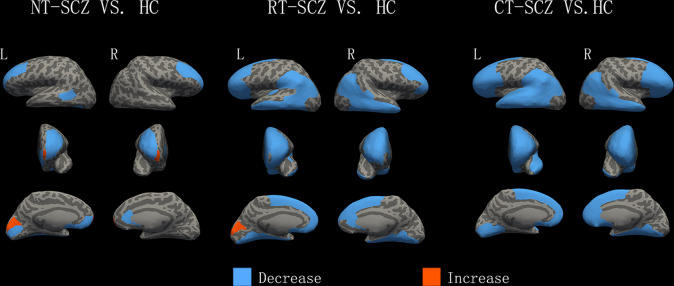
Differences in GM between patients with chronic schizophrenia and healthy controls. Greater cortical volume, surface area, or thickness in patients than in comparison subjects are indicated by red/warm color, and lower volume, surface area, or thickness are indicated by blue/cold color. L, left hemisphere; R, right hemisphere; HC, healthy controls; NT-SCZ, never treated schizophrenia patients; RT-SCZ, risperidone-treated schizophrenia patients; CT-SCZ, clozapine-treated schizophrenia patients.
